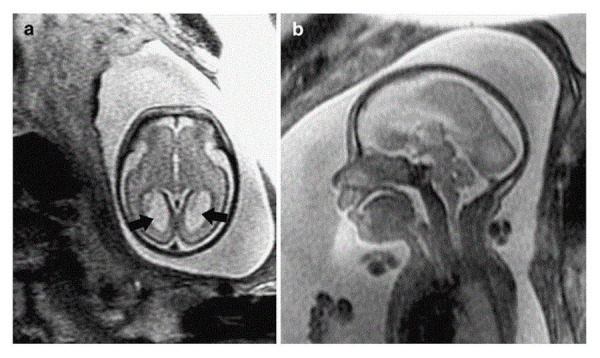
Colpocephaly Panel A is an axial T2-weighted single shot fast spin echo (SSFSE) image demonstrating colpocephaly (arrows) in a 26-week gestational age fetus. Panel B is a sagittal T2-weighted SSFSE image demonstrating absence of the corpus callosum. Image reprinted without any changes from Glenn, et al. under the creative common license.
